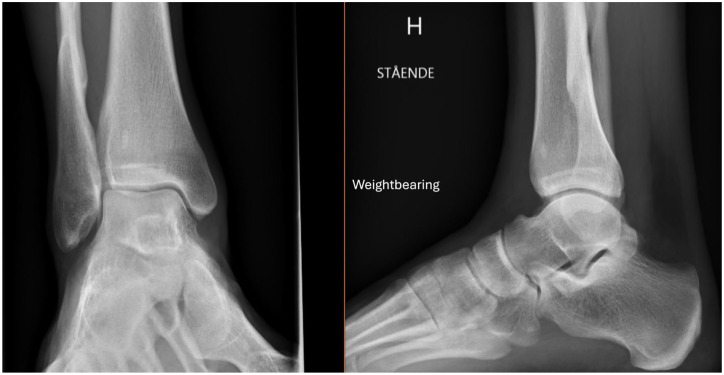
Weightbearing radiographs at the 12-month follow-up showing fracture healing and maintained ankle joint congruency.
